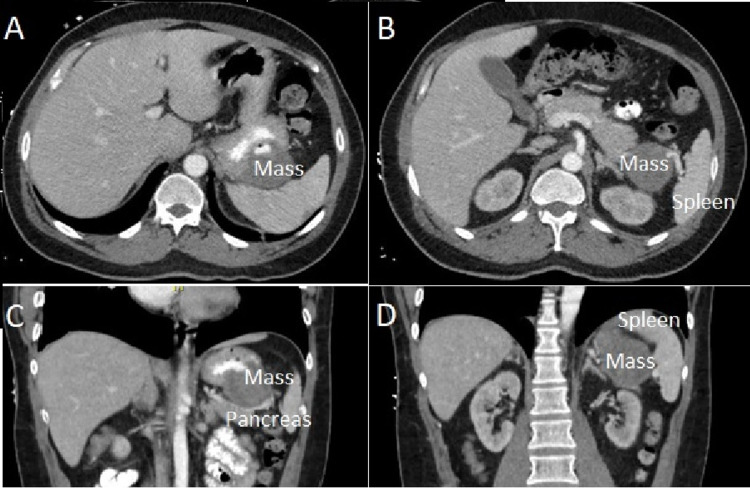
CT imaging of the gastric mass. A) Axial cut of the superior abdomen, demonstrating ulceration of the mass and proximity along the superior posterior gastric wall. B) Axial cut of the superior abdomen, demonstrating proximity of the mass to the splenic hilum. C) Coronal cut of the abdomen, demonstrating proximity of mass to pancreatic tail. D) Coronal section of the abdomen demonstrating proximity to the splenic hilum.
